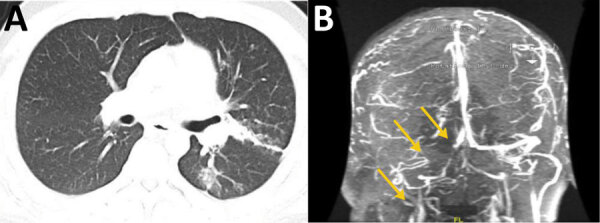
Radiographic findings from 25-year-old Hispanic man from New Mexico, USA (case 5), in multicentric case series of coccidioidal otomastoiditis. A) Computed tomography scan of the chest with contrast, showing septic emboli of the left lower lung lobe and lingula with developing pneumonia. B) Magnetic resonance venogram, showing stable thrombus of the right jugular bulb, right sigmoid and transverse sinuses, and partially occlusive thrombus in the anterior superior sagittal sinus, seen by lack of enhancement (arrows) compared with the left side.
